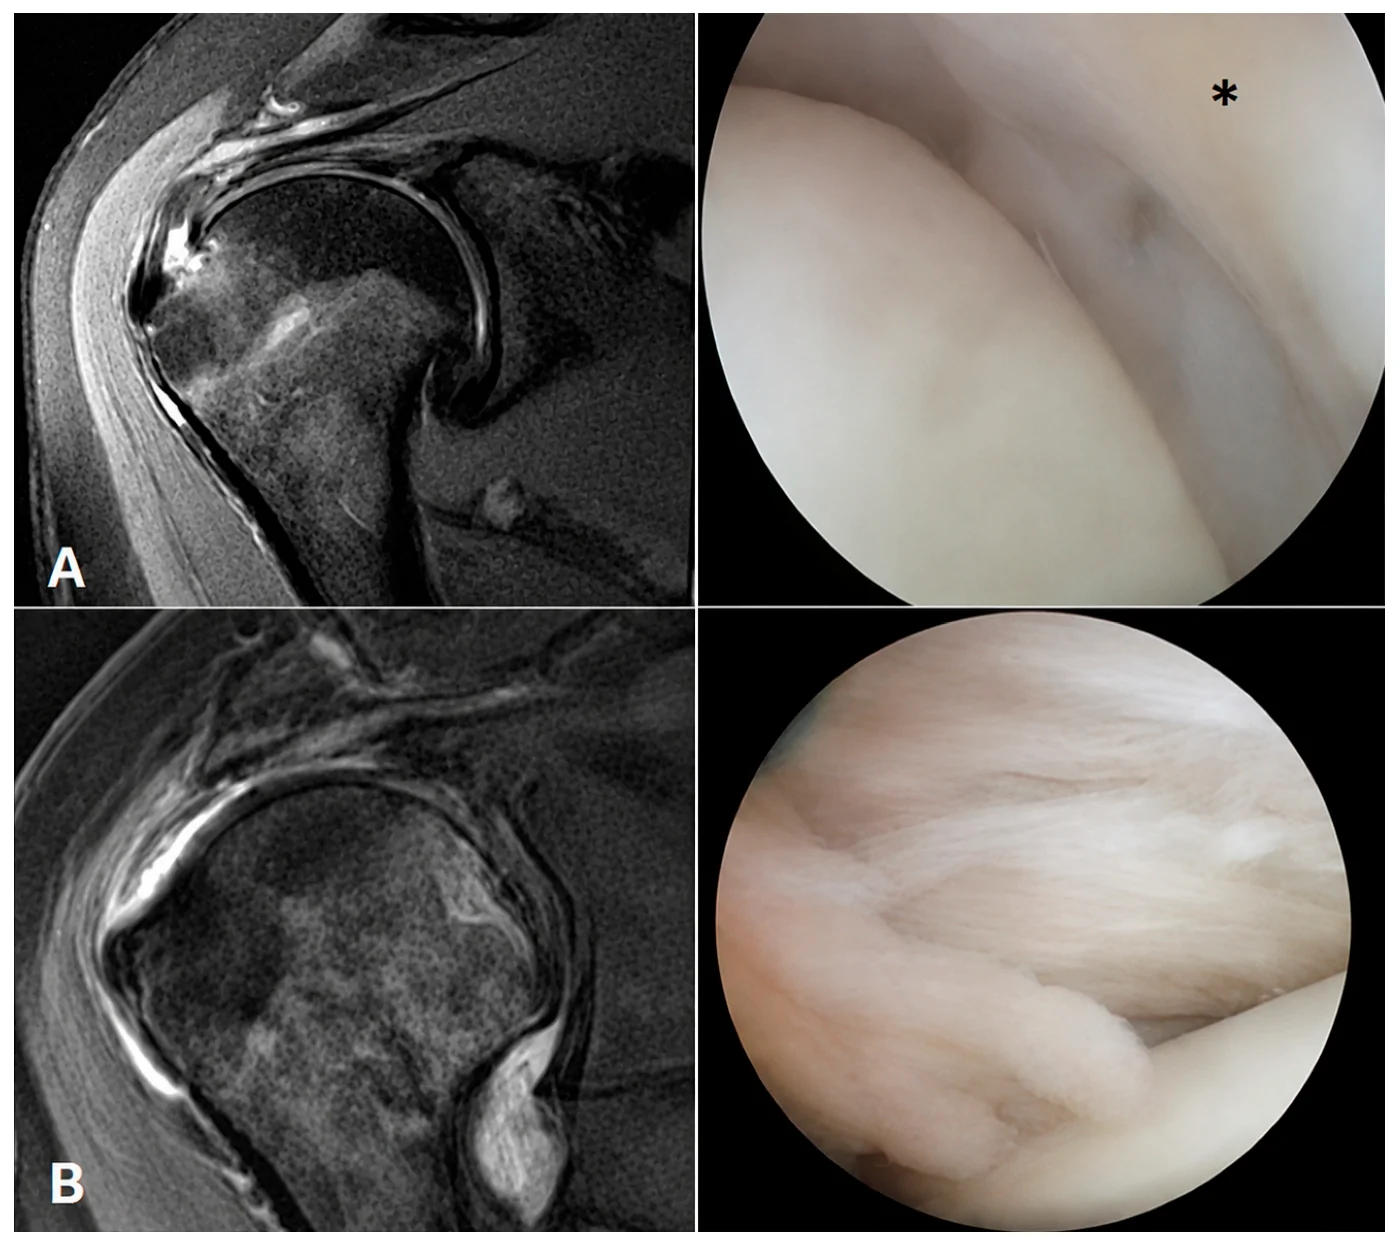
Representative arthroscopic (right) and MRI (left) images of the right shoulder. (A) The rotator cable was visible on arthroscopy but not identifiable on MRI. (B) The rotator cable was not visible on either arthroscopy or MRI. * indicates the rotator cable.
